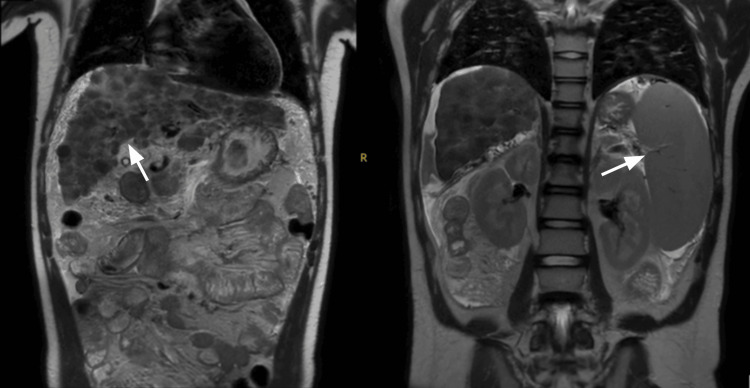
MRI scan of the abdomen showing irregular nodular surface of the liver, with hepatomegaly (left) and splenomegaly (right)
